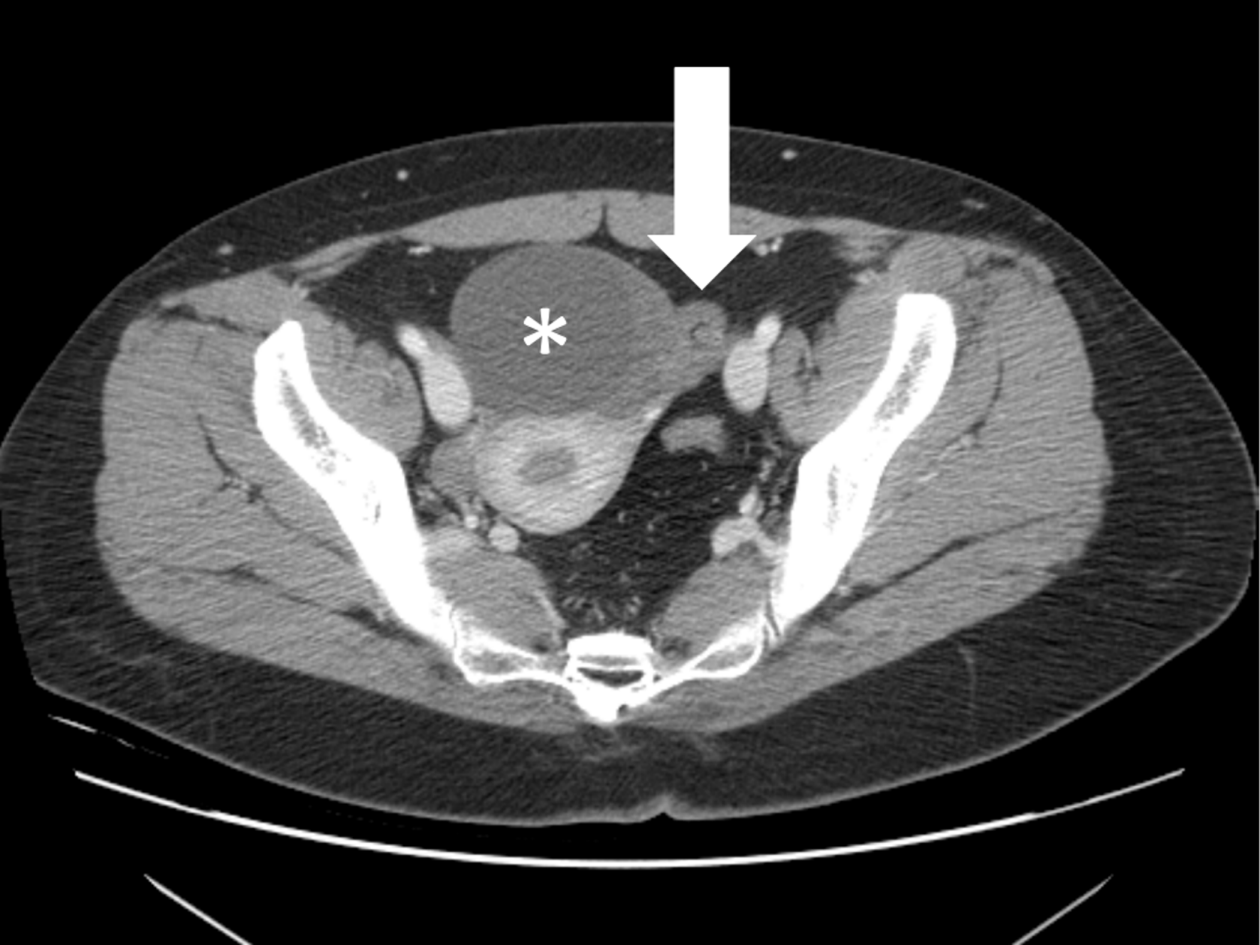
A 29-year-old woman with torsed left ovarian mature cystic teratoma. Axial contrast-enhanced CT scan shows a thickened pedicle (arrow) between a left ovarian cystic mass (asterisk) and the left uterine cornu with helical swirling appearance, suggestive of a whirl sign.
